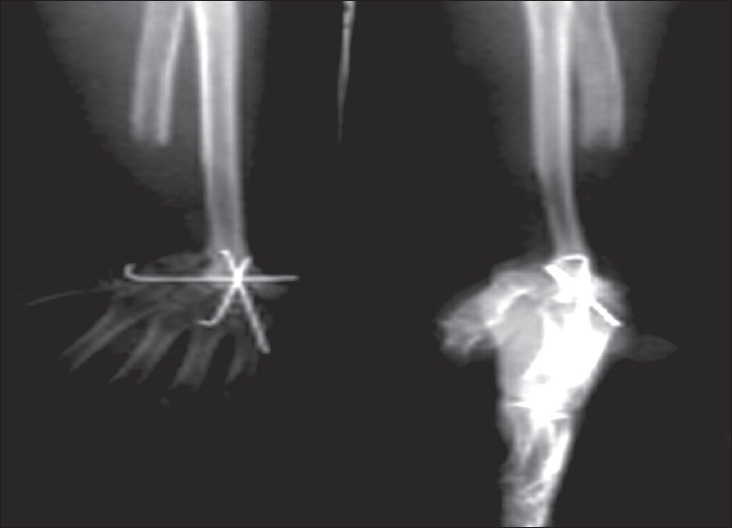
A.P. & Lateral x-ray at a two year follow up of a grade 3 lesion treated with excision and wrist arthrodesis by centralization of the carpus on the ulna. Good fusion seen even with K wire stabilization with advantage of retained prono-supination
